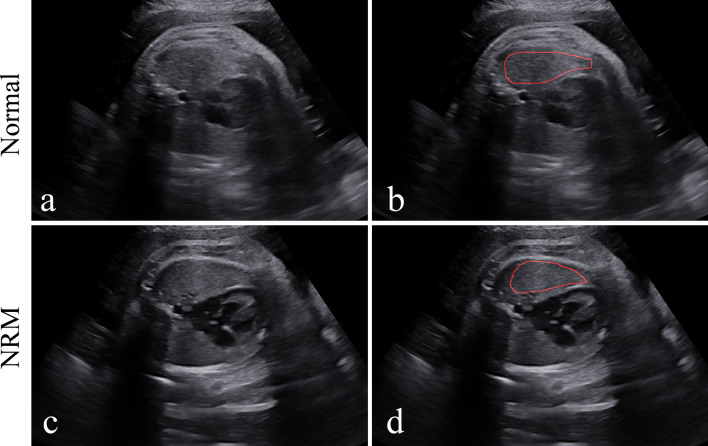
Examples of NRM and normal fetal lung ultrasound images and manual delineation. a An NRM fetal lung ultrasound image. b A normal fetal lung ultrasound image. c and d are the manual delineations of the ROIs
